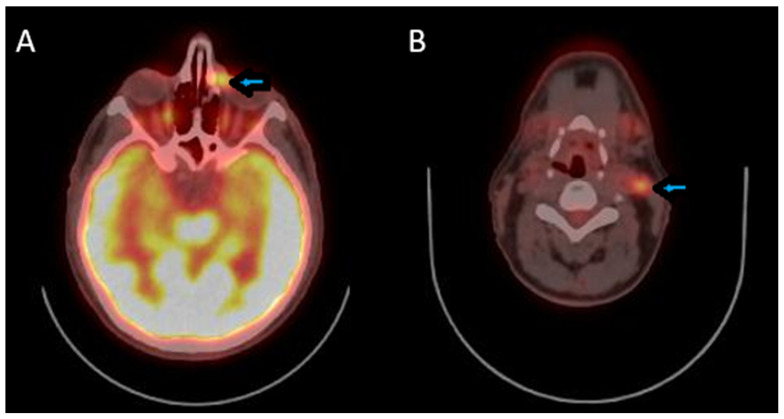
(A) PET-CT scan indicating a hypermetabolic lesion (arrow) in the extraconal compartment of the left orbital cavity, consistent with metastatic disease. (B) PET-CT showing hypermetabolic lymph node (arrow) in the left lateral cervical region, levels IIA–IIB, suggestive of metastatic involvement.
